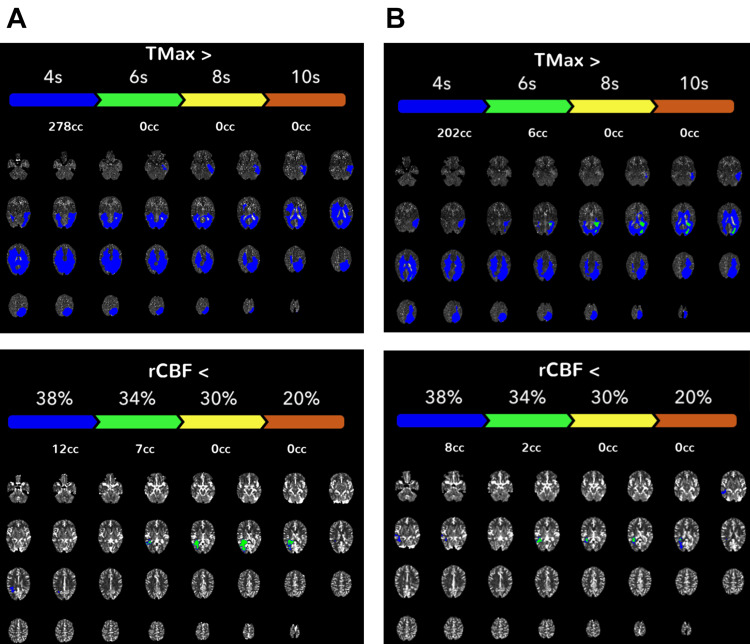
CT perfusion studies at six-month follow-up demonstrating improved hemodynamics compared with initial presentation A) Baseline time-to-maximum (Tmax) and regional cerebral blood flow (rCBF) pre-acetazolamide study in the left parietal temporal lobe. B) Tmax and rCBF after acetazolamide administration.
